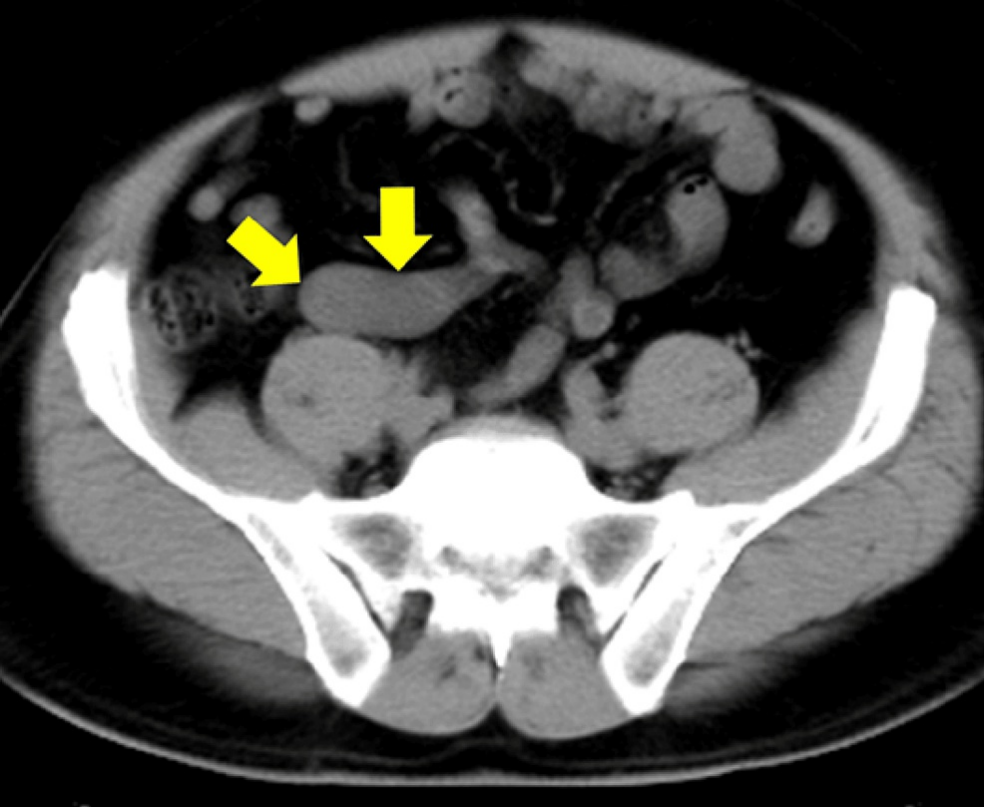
Plain computed tomography 12 years ago (axial image). A plain CT scan obtained 12 years ago showed that the appendix had enlarged to 20 mm (>13 mm) in size, and although wall calcification was not observed, the increase in the concentration of surrounding adipose tissue was not noticeable. These findings suggested appendiceal mucinous neoplasm.
